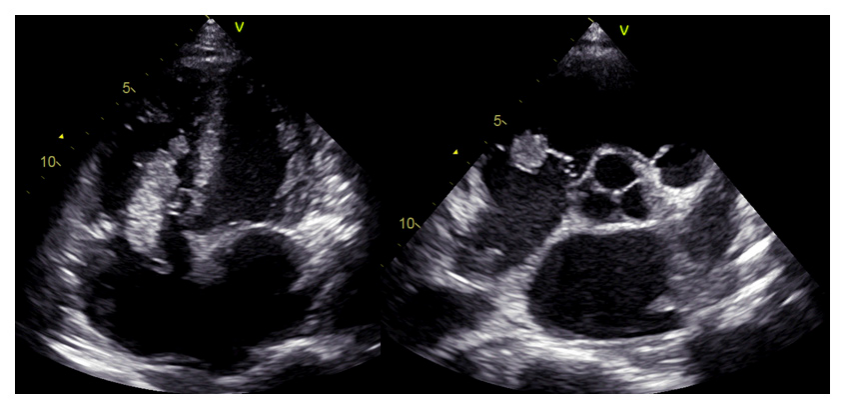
Transthoracic echocardiogram (TTE) showing protruded thrombus in the right atrium passes through the tricuspid valve.
